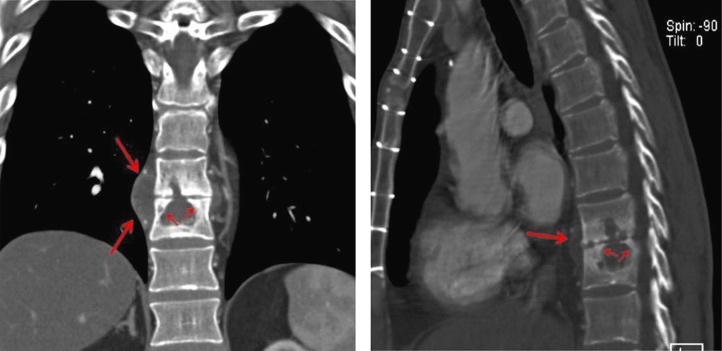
A coronal (left) and a lateral (right) reformation from the CT scan better demonstrate the paraspinal swelling (long arrows) and the collapsed disc, the lytic defects in the vertebra from tuberculous osteomyelitis, and the healing sclerosis postantibiotic treatment (short arrows).
